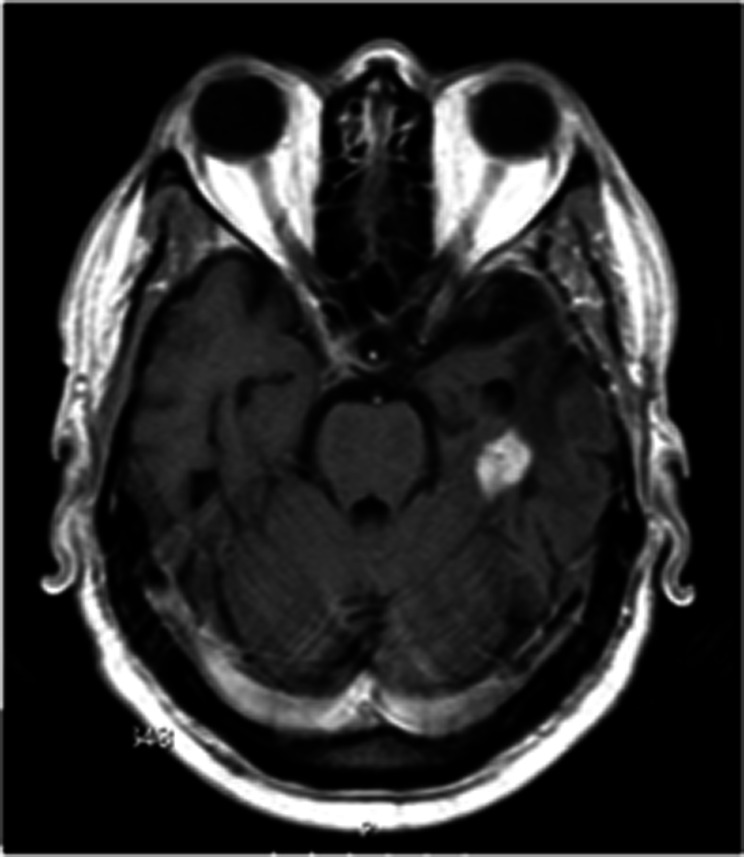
Axial T1 weighted gadolinium enhanced MRI scan showing gadolinium-enhanced nodular lesion in the left temporal lobe.
